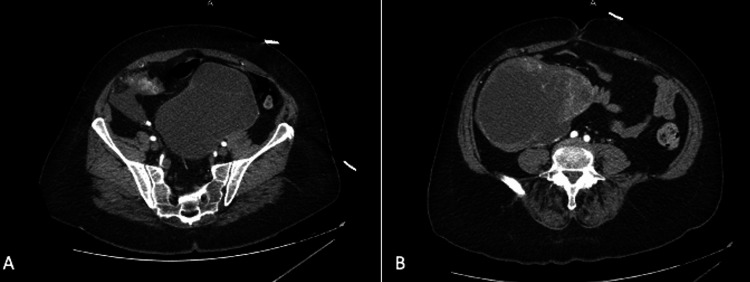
Axial images of CT scan showing bilateral ovarian masses. A) Depicts a serous cystadenoma with chronic inflammation and early fibrosis. B). Depicts the poorly differentiated thyroid carcinoma arising from a malignant struma ovarii. CT, computed tomography
